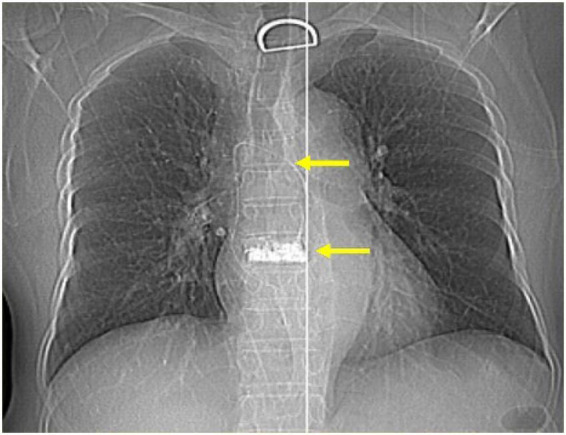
X-ray shows the high-density shadow from the eighth thoracic vertebrae to the right lung (yellow arrows).
